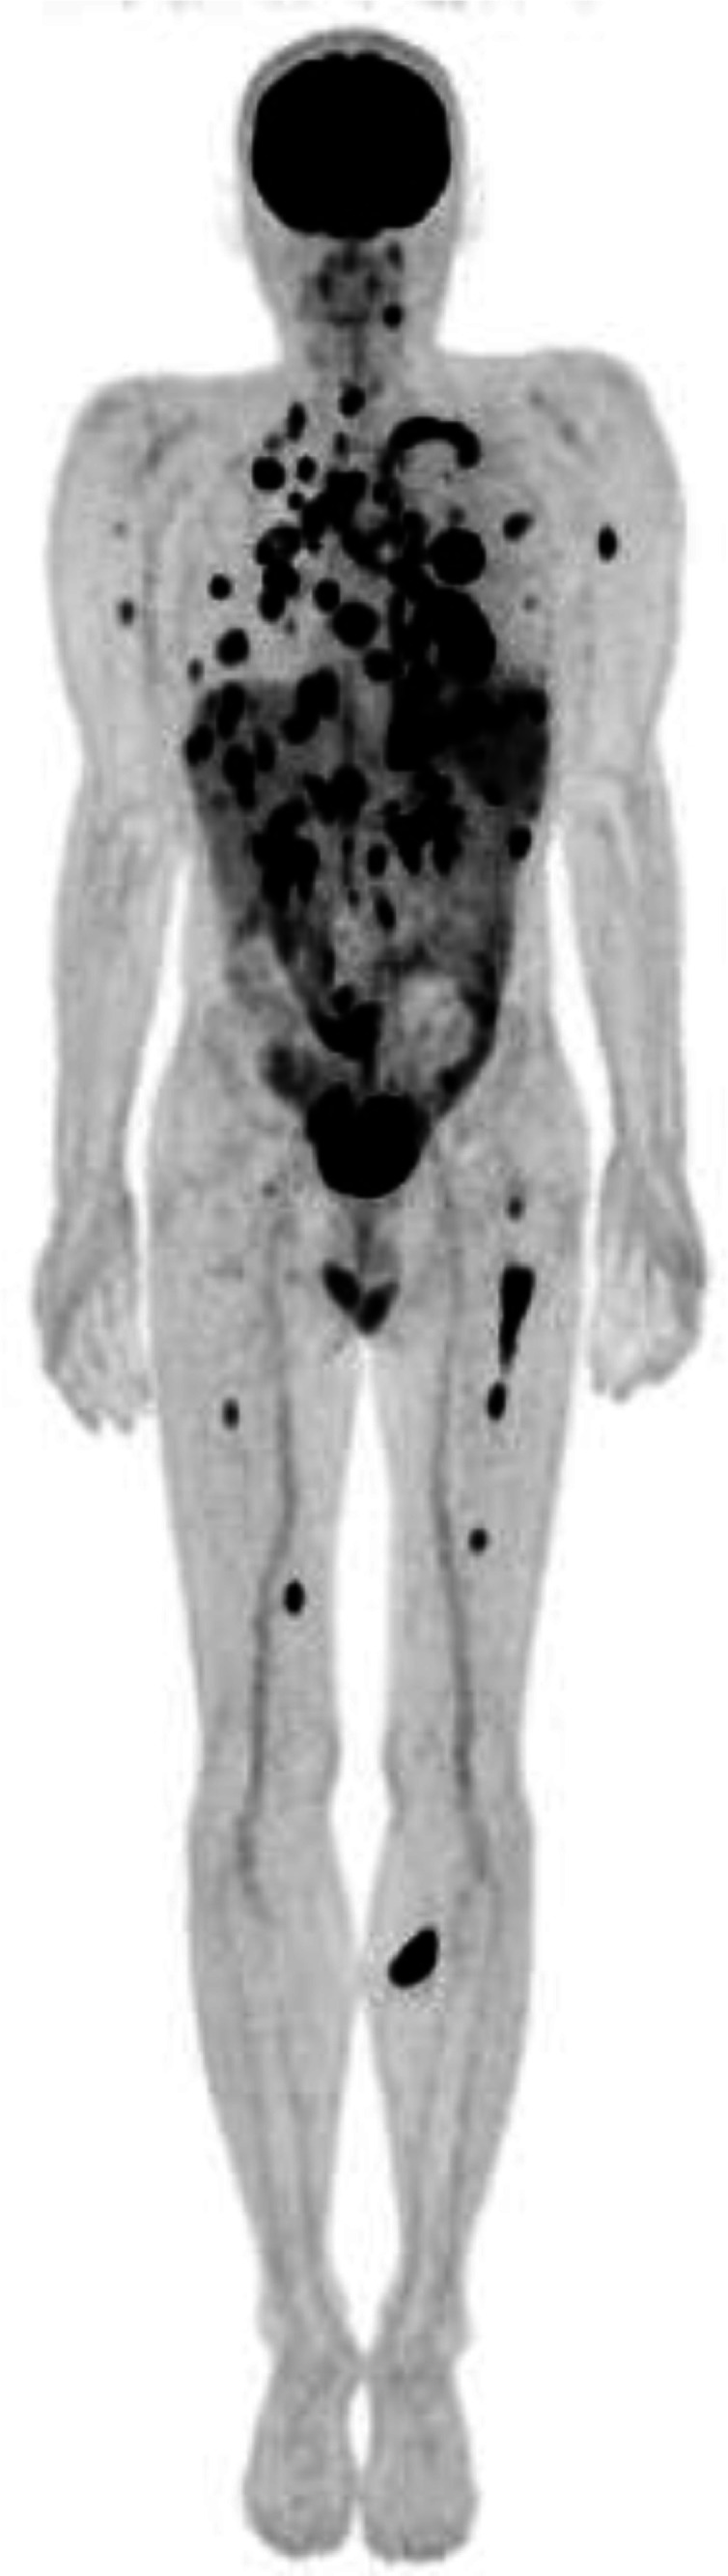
PET-CT image at 9 months after initial presentation. The maximum intensity projection (MIP) image shows multiple areas of FDG uptake, consistent with metastases.
